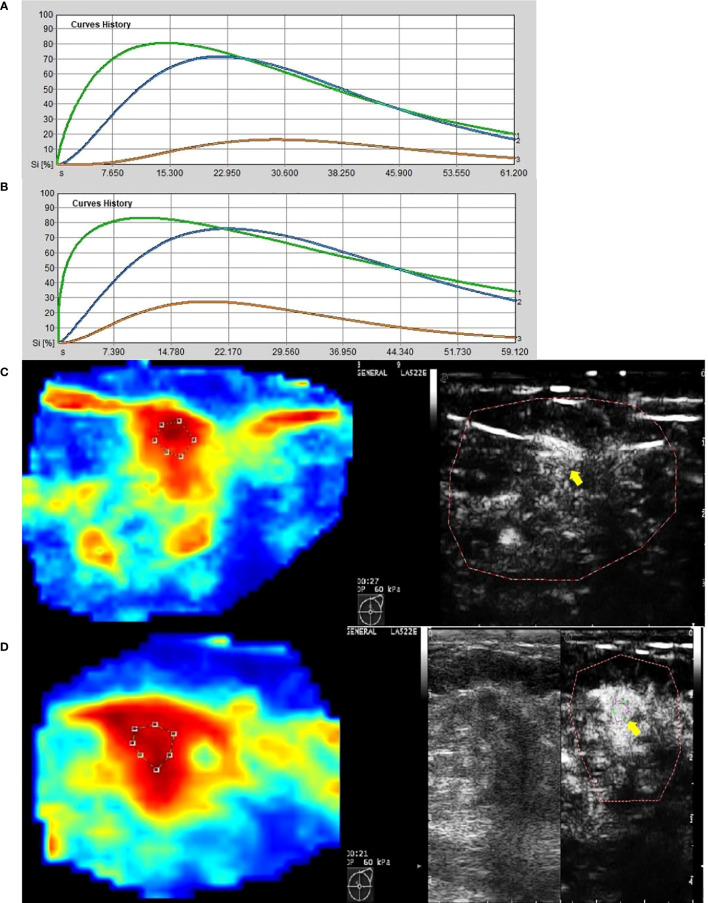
Images from a patient of non-response to NAC. (A) At baseline before NAC, contrast-enhanced ultrasound imaging showed a time-intensity curve generated according to regions of interest. The green line represents ROI 1 (the greatest enhancement area in central region of tumor), the blue line represents ROI 2 (the greatest enhancement area in edge of tumor), the yellow line represents normal breast tissue. (B) After the first cycle of NAC, neither ROI 1 nor ROI 2 declined significantly. (C) At baseline before NAC, the circle represents ROI 1, and the red area means rich blood supply; the right image was gray-scale which correspond to the left one, and the yellow arrow referred to ROI 1. (D) After the first cycle of NAC, the circle represents ROI 1, and the red area expanded, instead of shrunk; the right image was gray-scale which correspond to the left one, and the yellow arrow referred to ROI 1.
